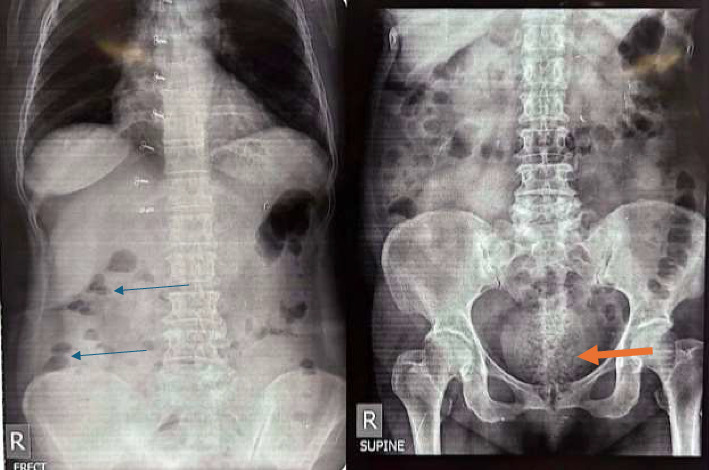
X-ray of abdomen and pelvis showing bowel loaded with stool on the rectal area (orange arrow) and on supine positives, 3-4 air fluid levels were visible suggesting large bowel obstruction.
